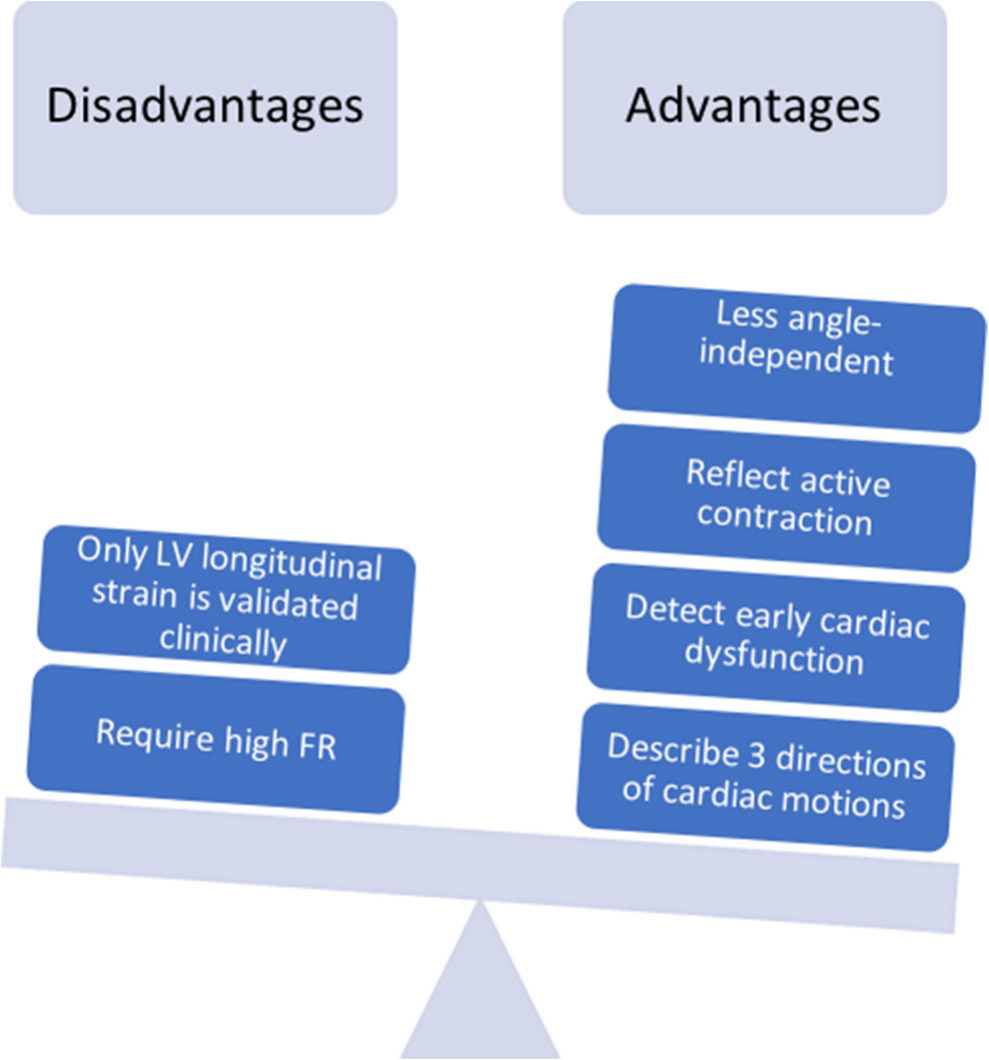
Speckle tracking echocardiography advantages. LV, left ventricular; FR, frame rate
